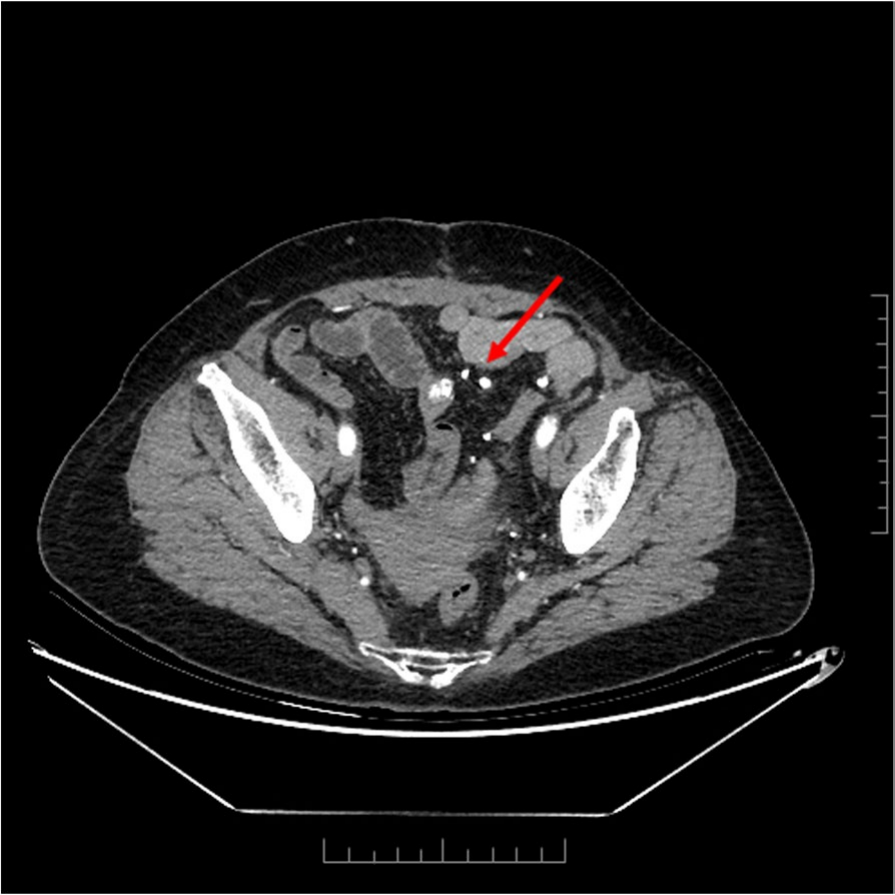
Abdominal CT-scan imaging: multiple new calcified masses found around the descending colon and the anastomotic, which showing irregular reinforcement, suspicious for metastasis (arrows)
